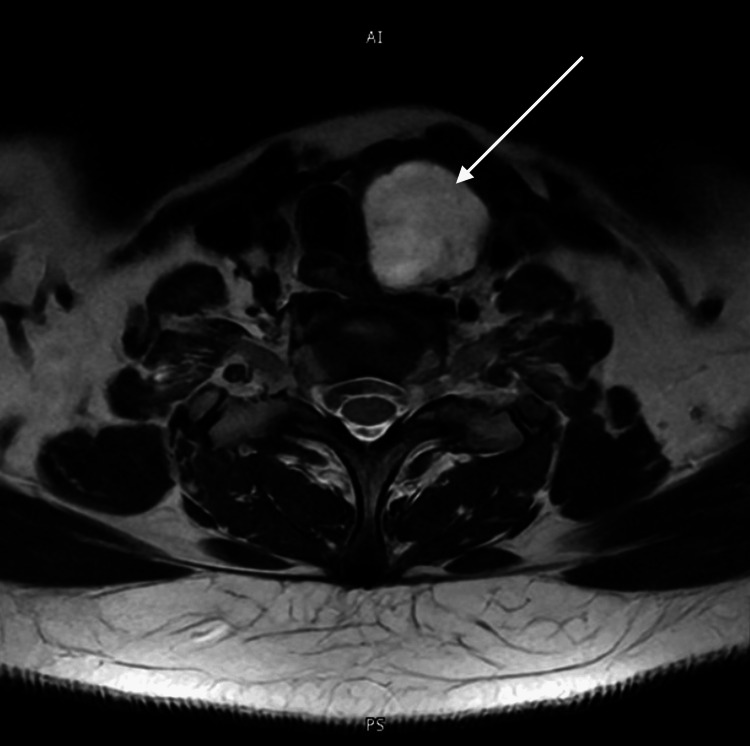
Cervical magnetic resonance imaging, T2-weighted axial sequence. Cervical MRI, T2-weighted axial sequence. The arrow highlights the hyperintense dominant nodule in the left lobe, indicative of cystic necrosis. The empty right thyroid bed is occupied solely by adipose tissue.
